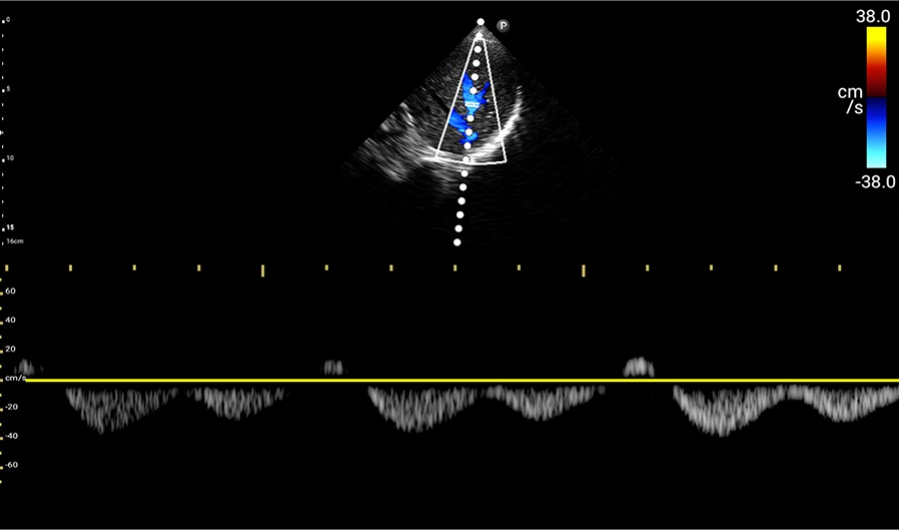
Pulsed-wave Doppler tracing of the HV in cardiac preset. HV, hepatic vein
